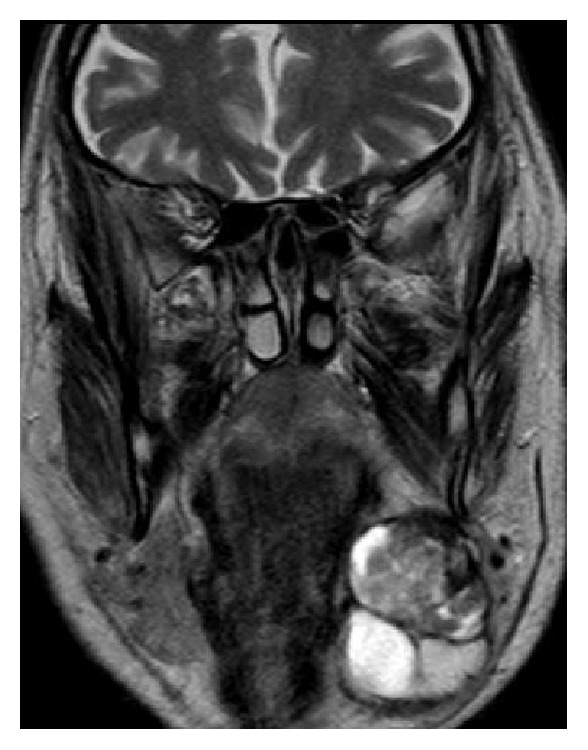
Preoperative MRI, coronal T2-W: 5 × 4 × 3 cm mass originating from the left submandibular gland. Two different multilobed neoformations with colliquate areas (black square and black trapezius) delimited by a thick pathological peripheral tissue are clearly visible.
